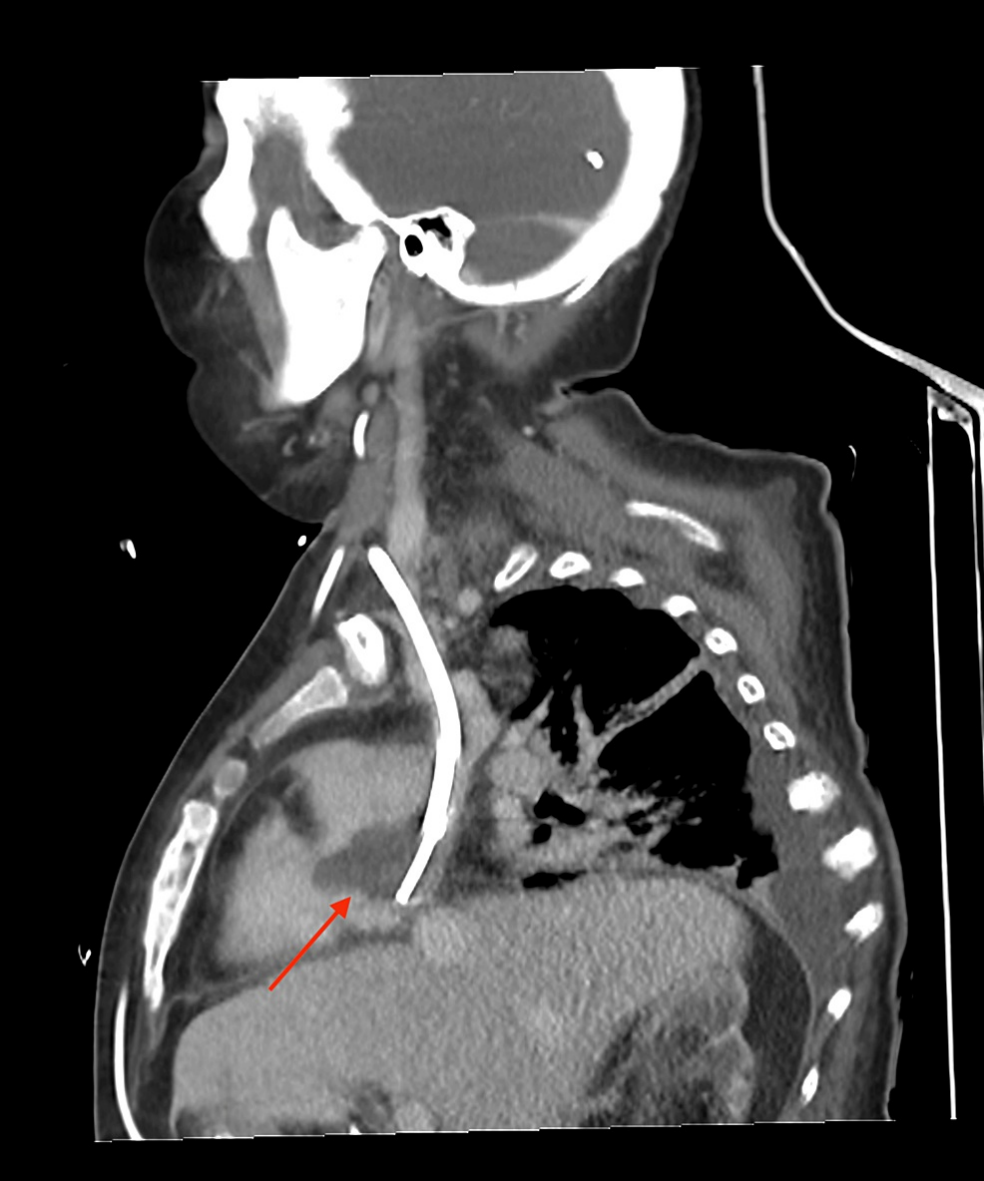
CT scan of the chest (sagittal view) Chest computer tomography shows the mass at the tip of the dialysis catheter into the right atrium (red arrow).
